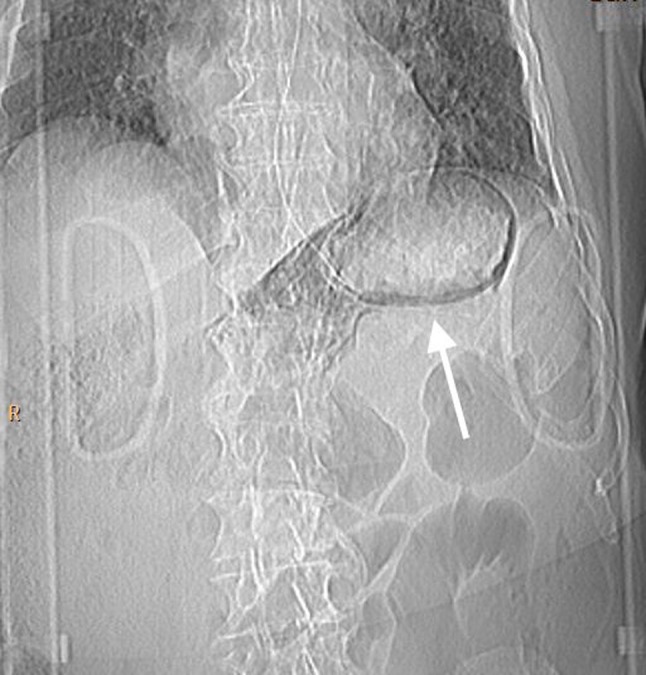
Topogram preliminary to the CT scan. Linear gas shadows in the upper left abdominal region (arrow), apparently round the stomach.
